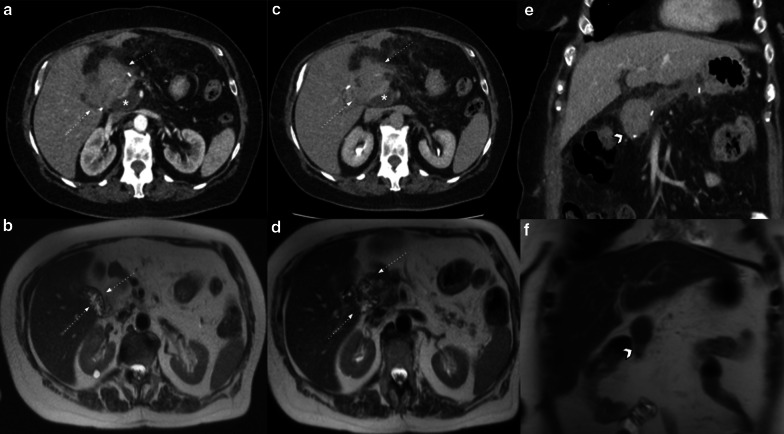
Collapsed loop vs tumor recurrence. The MRI role. 62-year-old female patient during routine follow-up after pancreaticoduodenectomy due to pancreatic adenocarcinoma three months early. (a, c, e) Axial and coronal view contrast-enhanced CT scan. Homogeneous fluid collection (asterisk) in the surgical bed and related to an ill-defined soft tissue enhancing image with peripheral fat stranding associated (dotted arrows). Coronal view denotes a pseudo-mass image (arrowhead in c). Collapsed loop versus inflammatory changes were suggested as possible diagnosis, so strict control was indicated. (b, d, f) MRI performed two months later. Prior collection complete resolution. The ill-defined image related to the hepatic hilum is now better defined as the collapsed loop due to their fluid content and intestinal folds (dotted arrow). Coronal view denotes the blind portion of the afferent loop as the previously described pseudo-mass image (arrowhead in f)
